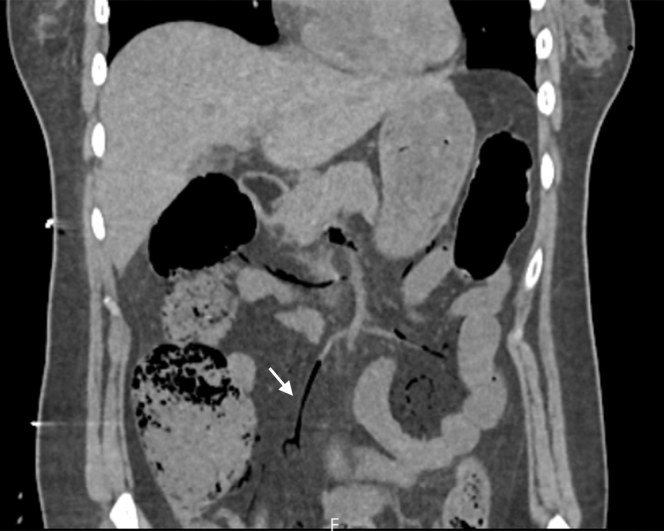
Coronal abdominal computed tomography: mesenteric venous pneumatosis (arrow, superior mesenteric vein branches). Linear gas tracks in superior mesenteric vein branches extended porto-mesenteric venous gas beyond the hepatic veins, ruling out isolated venous gas and suggesting more extensive gastrointestinal involvement.4.Axial abdominal CT: submucosal gas in the ascending colon (colonic pneumatosis intestinalis) was the critical high-risk feature—this finding, combined with PMVG and hypotension, strongly predicted bowel ischemia, prompting urgent surgical consultation (Fig 4).
